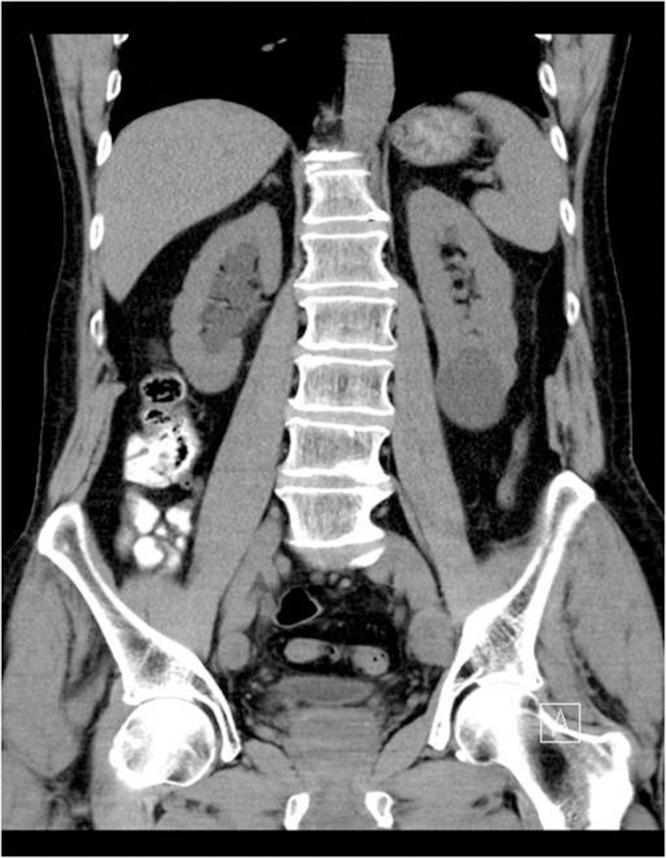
Computed tomography showing right hydronephrosis
